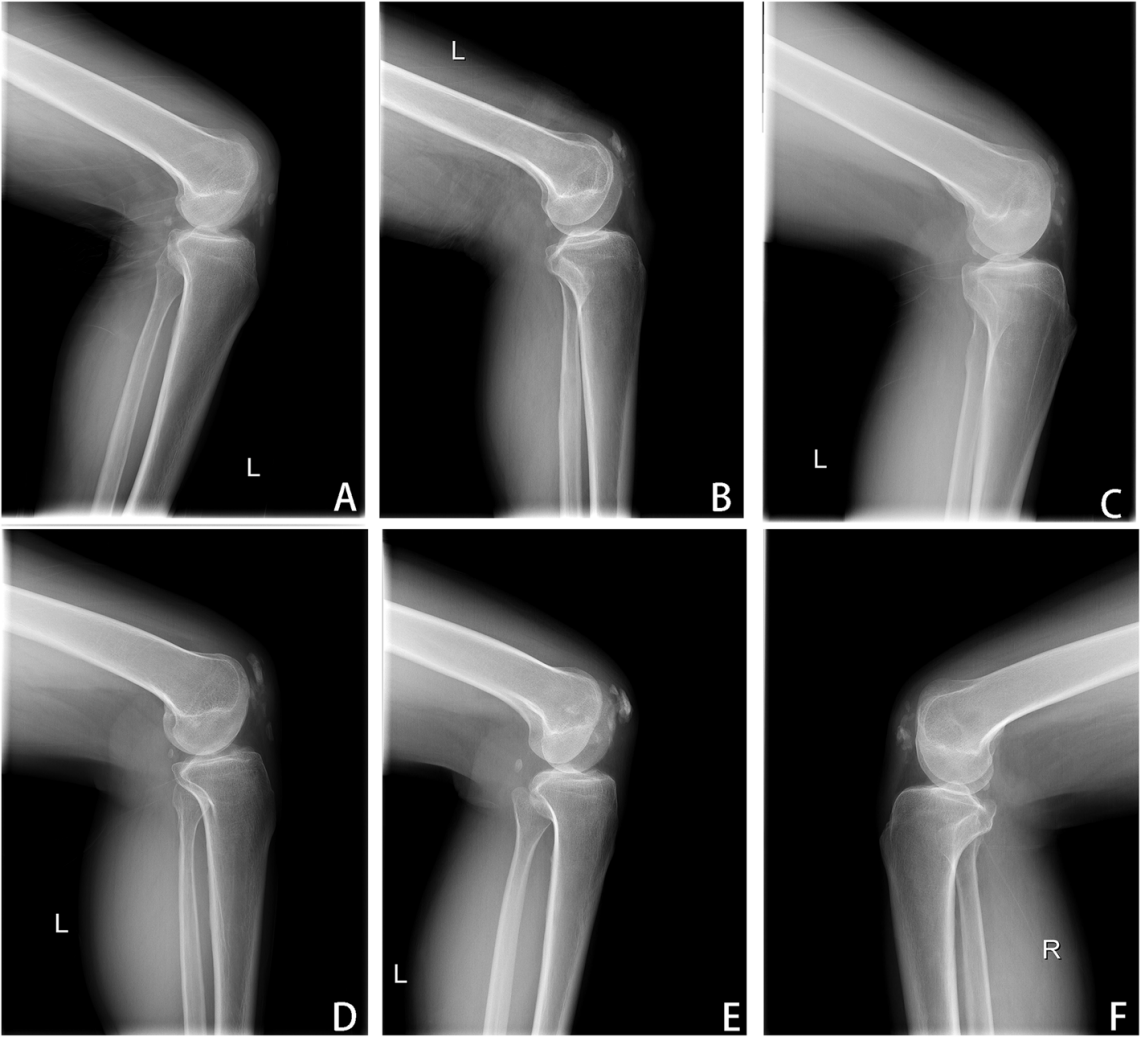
Postoperative lateral knee radiographs showed patients developed calcification after total patellectomy at the final follow-up
